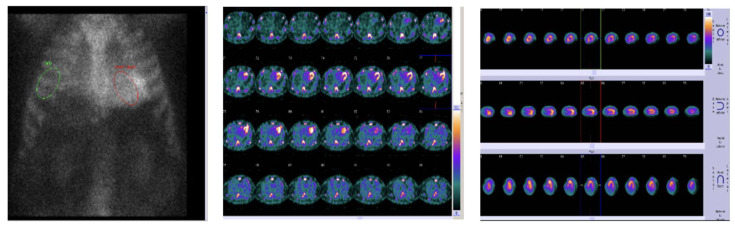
PYP scan for patient with suspected cardiac amyloidosis showing increased heart/lung ration with grade III uptake on SPECT images.
